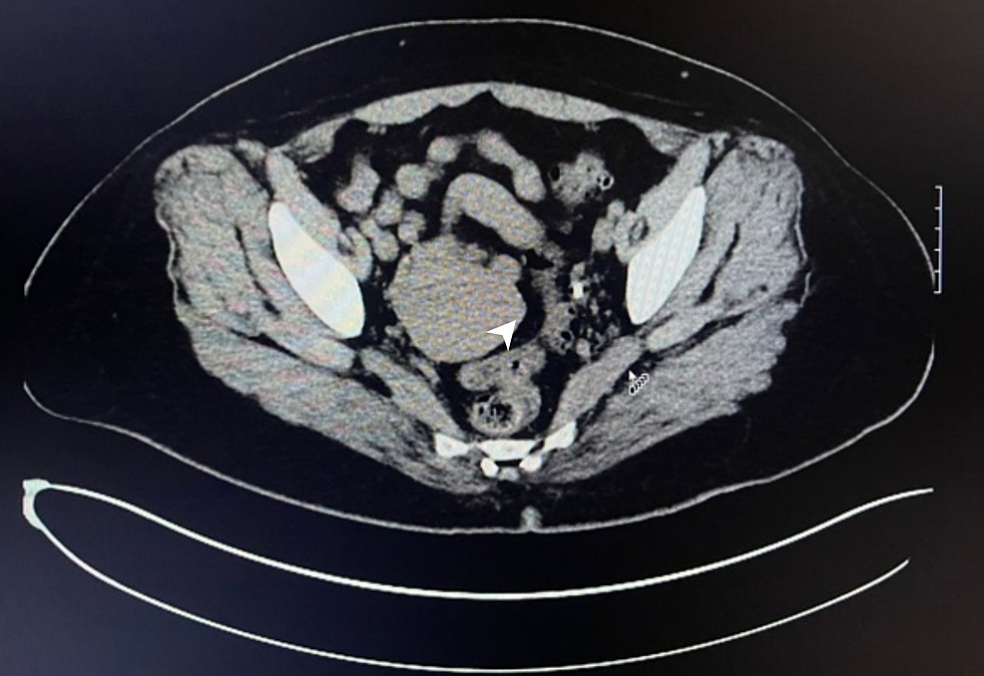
CT abdomen and pelvis after treatment showing resolution of mass (location indicated by a white arrowhead) CT: computed tomography
